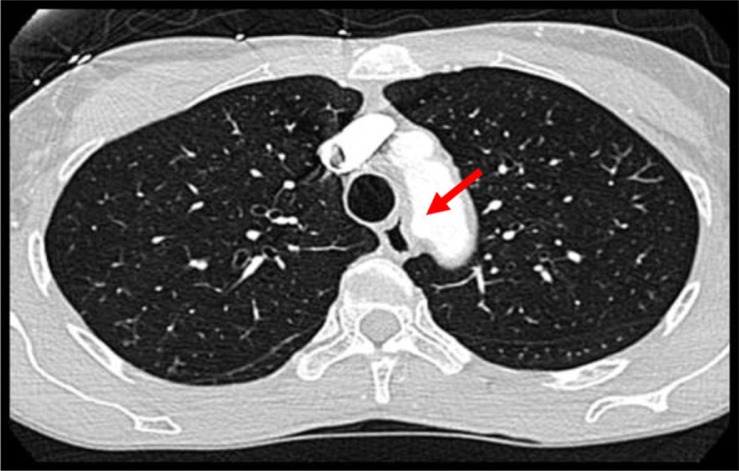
CT chest with contrast showing the origin of the aberrant right subclavian artery (red arrow) causing extrinsic compression of the esophagus.
